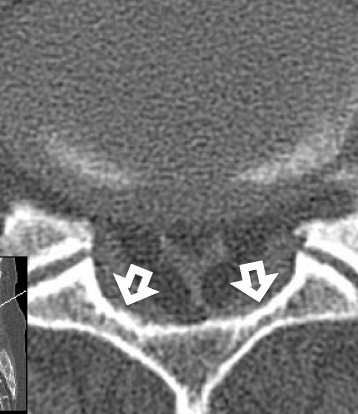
Saucerization of laminae on computed tomography. Saucerization of laminae is defined as a dome-formed spinal canal with thinness of the vertebral lamina (arrows)
